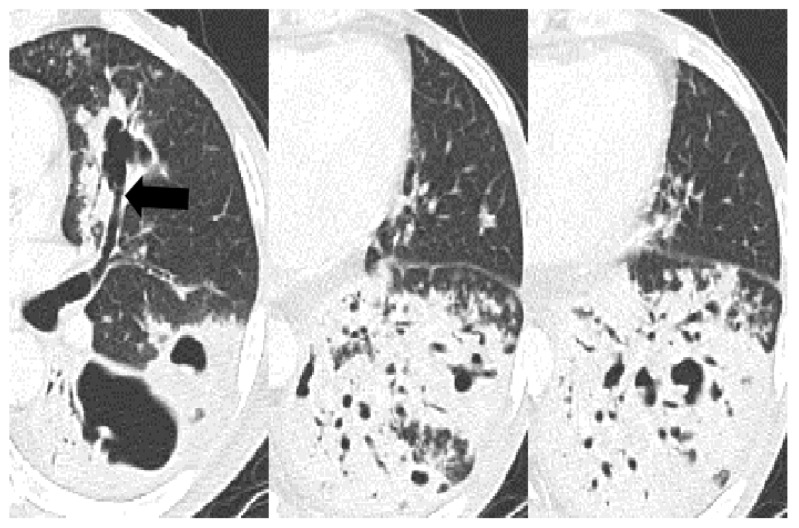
A 55-year-old male with pulmonary tuberculosis and uncontrolled diabetes (HbA1C, 8.30%). Cylindrically dilatated subsegmental bronchus in the superior lingula communicates with the cavity in axial CT scans (feeding bronchus sign, arrow). Multiple feeding bronchus signs and bronchial erosions within the consolidations are also noted in the left lower lobe. Dilated air bronchograms have bead-like nodules hanging from them.
